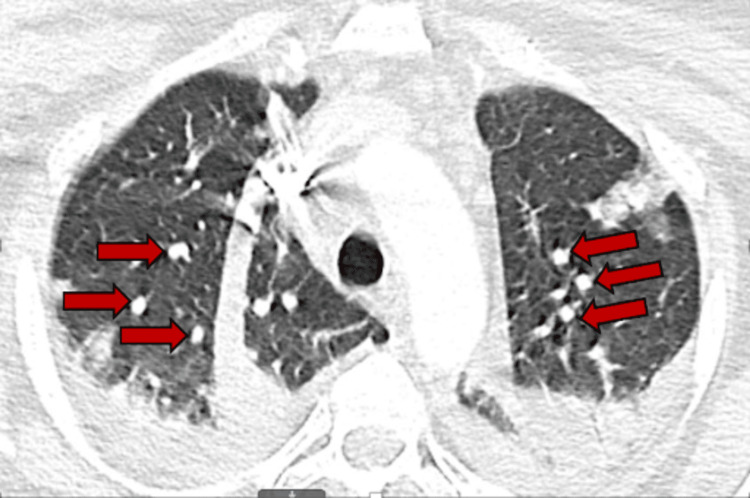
Computed tomography angiography revealed bilateral multifocal nodular infiltrates concerning for septic emboli.
